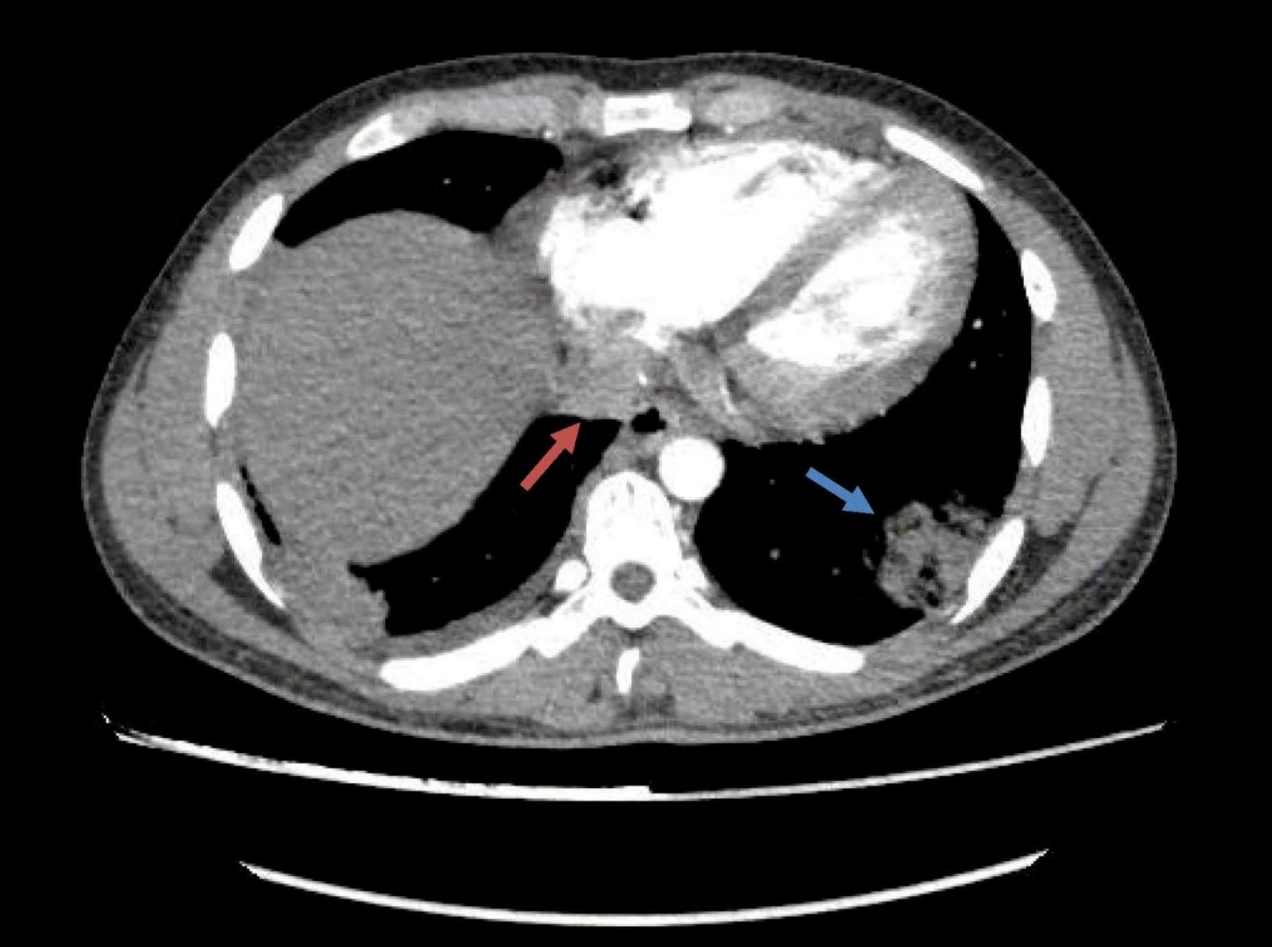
Contrast-enhanced computed tomography pulmonary angiogram. Red arrow shows a large filling defect in right atrium. Blue arrow shows dense consolidation in left lateral basal segment suggestive of infarct.
